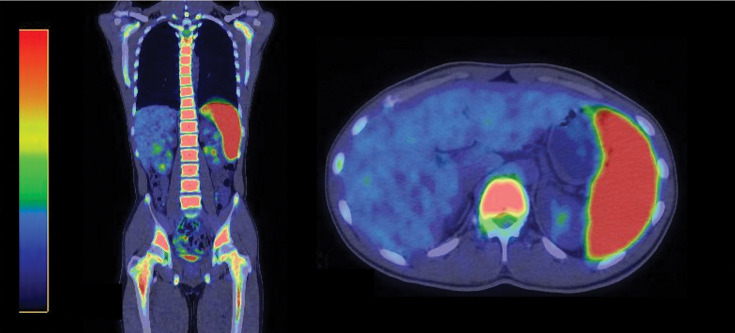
Positron emission tomography–computed tomography showed diffuse abnormal accumulation of fluorodeoxyglucose in the bone marrow. The splenomegaly rapidly worsened within 1 month, and abnormal fluorodeoxyglucose accumulation was observed in the spleen.
